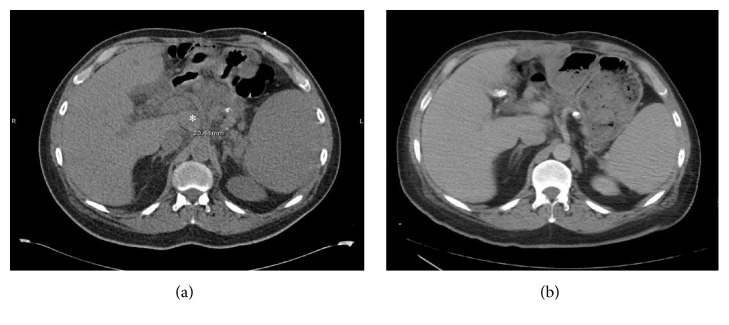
(a) CT without contrast. Pretreatment lymph node (*∗*) is 2 cm. (b) CT with contrast. Posttreatment lymph node on a comparable level is not visible.
